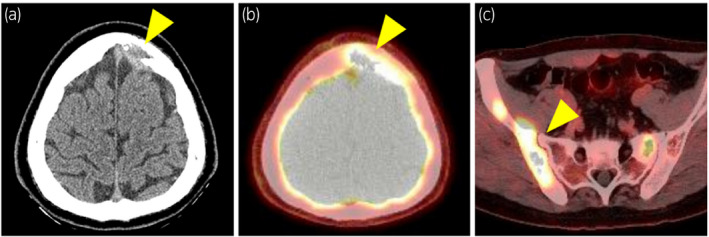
Imaging evaluation at tumor marker re‐elevation showed exacerbation of the bone metastatic lesions. (a) CT revealed progressive bone destruction of the frontal bone and an increase in soft tissue tumors (arrowhead). (b) PET showed high accumulation in the frontal bone lesions (arrowhead). (c) PET disclosed high accumulation in osteolytic lesions in the left iliac bone (arrowhead).
